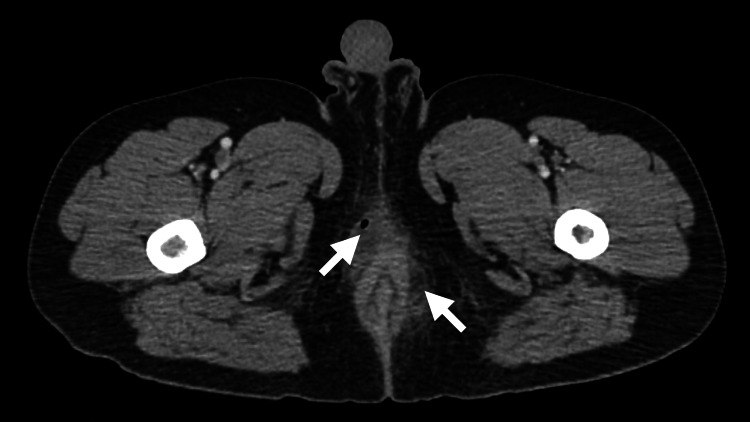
CT image of the perianal abscesses in the right anterior and left lateral regions.
